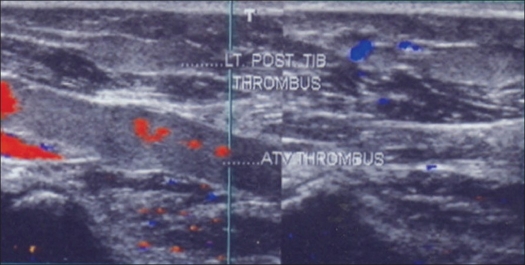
Ultrasound color Doppler showing thrombosed left anterior and posterior tibial vein
